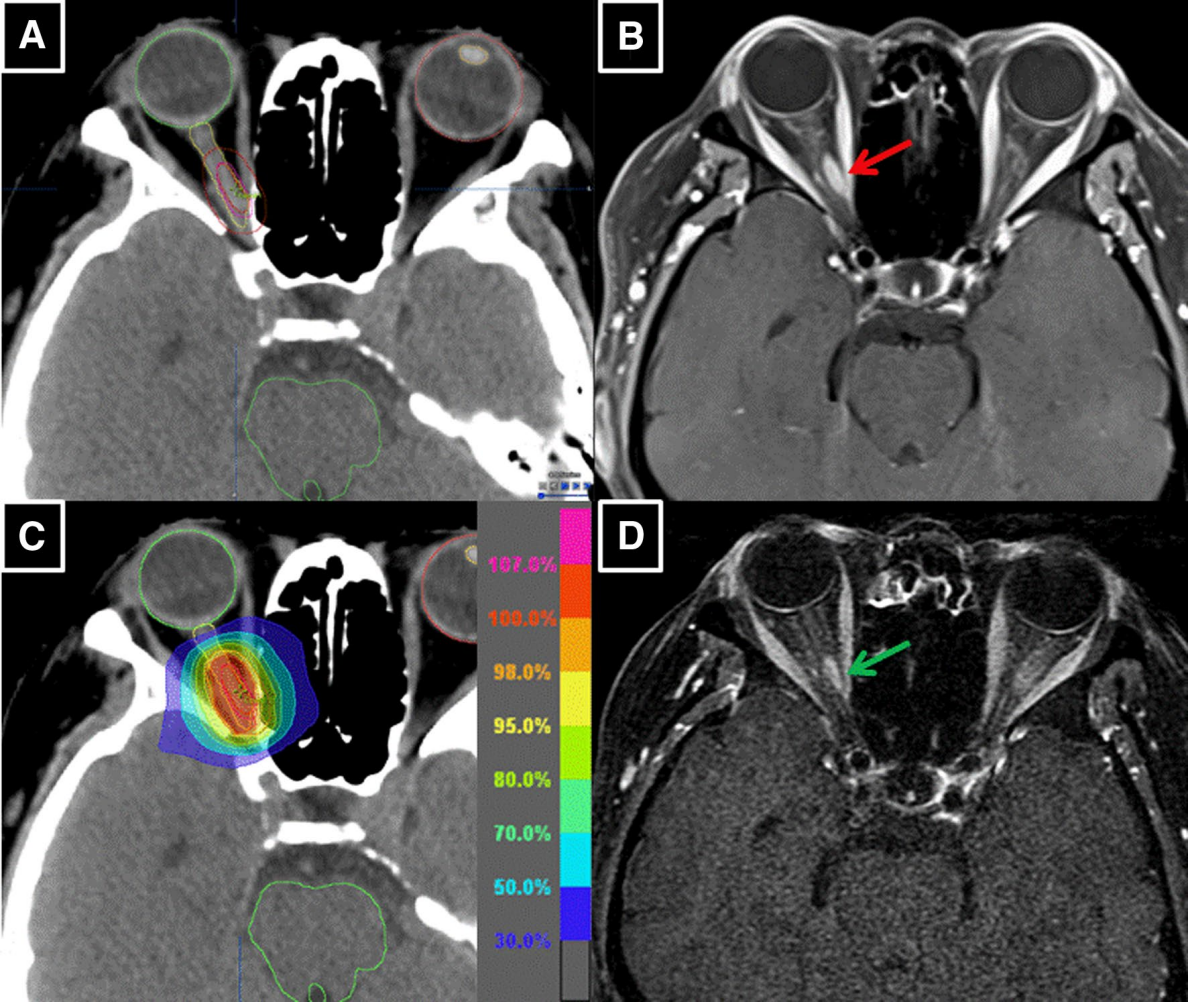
Patient #2 was a 57-year-old woman. Pretreatment computed tomography (CT) (a) and magnetic resonance imaging (MRI) showed a 13-mm-long, fusiform type of intraorbital tumor (red arrow) on the medial side of right optic nerve (b). She underwent IMRT of 54 Gy in 30 fractions over 43 days in January 2014 (c). MRI performed 46 months after IMRT revealed 39% reduction of the tumor volume (green arrow) (d)
